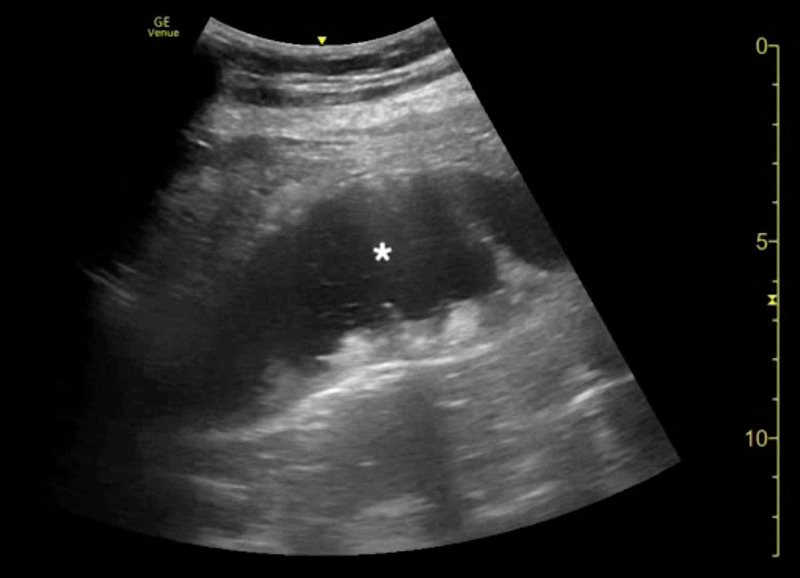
Point-of-care ultrasound of gastric volvulus post-nasogastric tube insertion demonstrating stomach decompression (asterisk).
